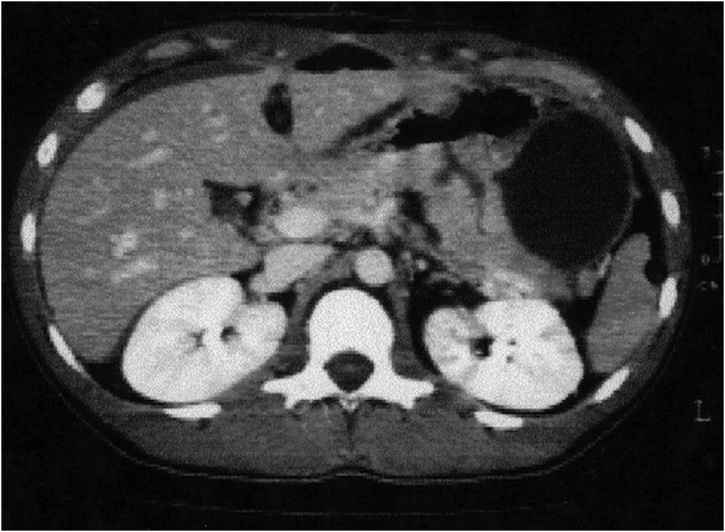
The CT-SCAN show the PG reconstruction and the pseudocyst that is treated with a percutaneous radiological drainage.
